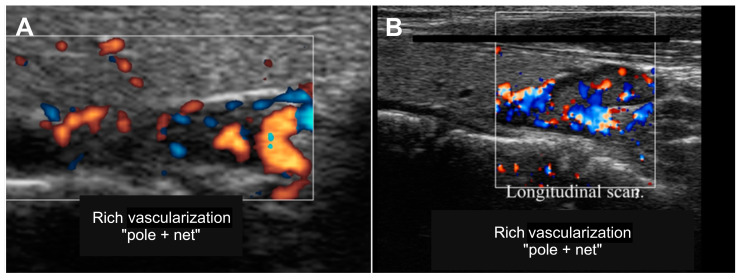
Ecocolor-Doppler US images of parathyroids (white rectangle), showing well-vascularized arterial pole (A) and arterial reticular pattern (B).
